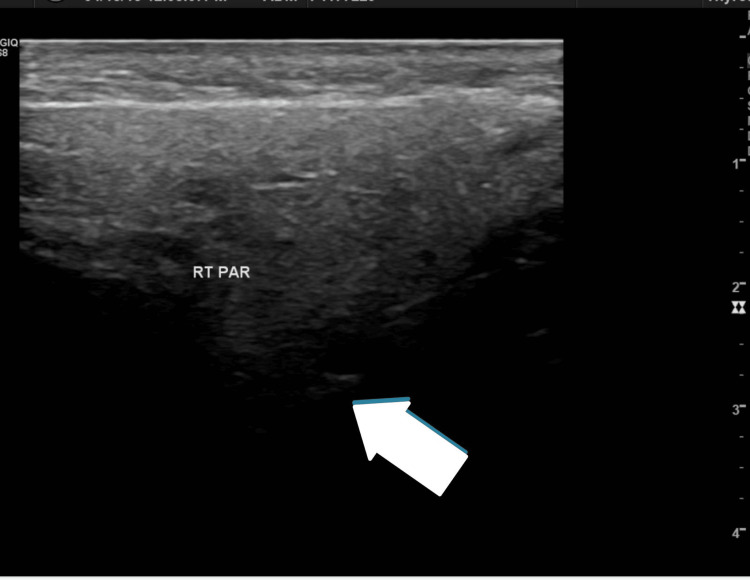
Ultrasound neck Bulky right parotid gland (4.2 cm x 2.5 cm, arrow) with homogeneous echotexture, no focal lesions, and small intra-glandular lymph nodes. RT PAR: Right Parotid
